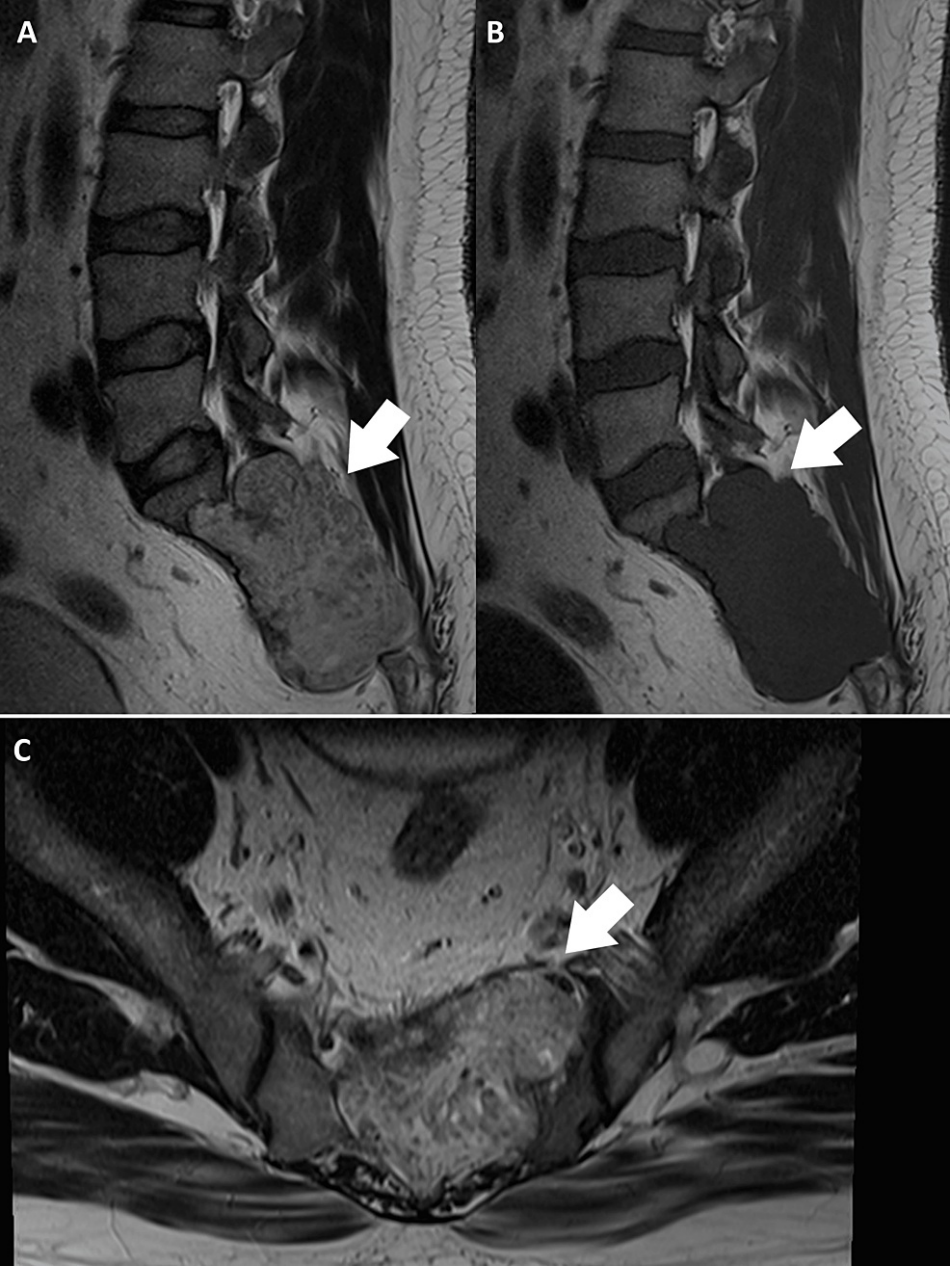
Lumbar magnetic resonance image (MRI): The presence of a moderately hypermetabolic soft tissue mass involving S1-S3 vertebral bodies with a presacral soft tissue component and measuring 6.96 × 5.12 cm (white arrow) strongly suggests a malignant process. (A) On T2-weighted images, high signal intensity can be seen. Destruction or erosion of the sacral bone, and possible extension into adjacent structures, can also be identified. (B) On T1-weighted images, the image appears isointense compared to the normal spinal cord. (C) On the coronal view, destruction or erosion of the sacral bone can be seen.
